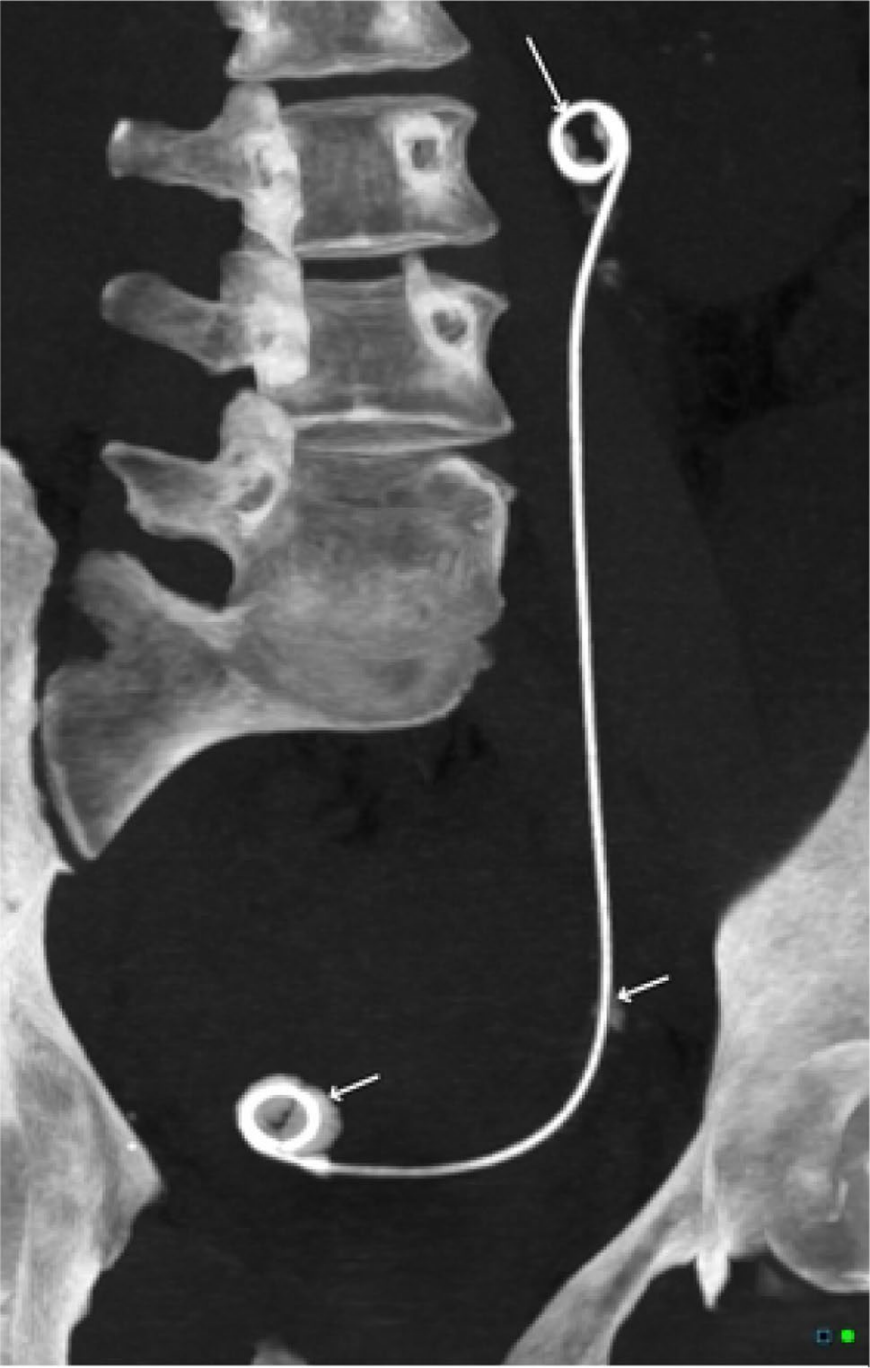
A coronal CT image of a ureteric stent in situ showing encrustations. Case courtesy of Dr Chris O’Donnell, Radiopaedia.org
